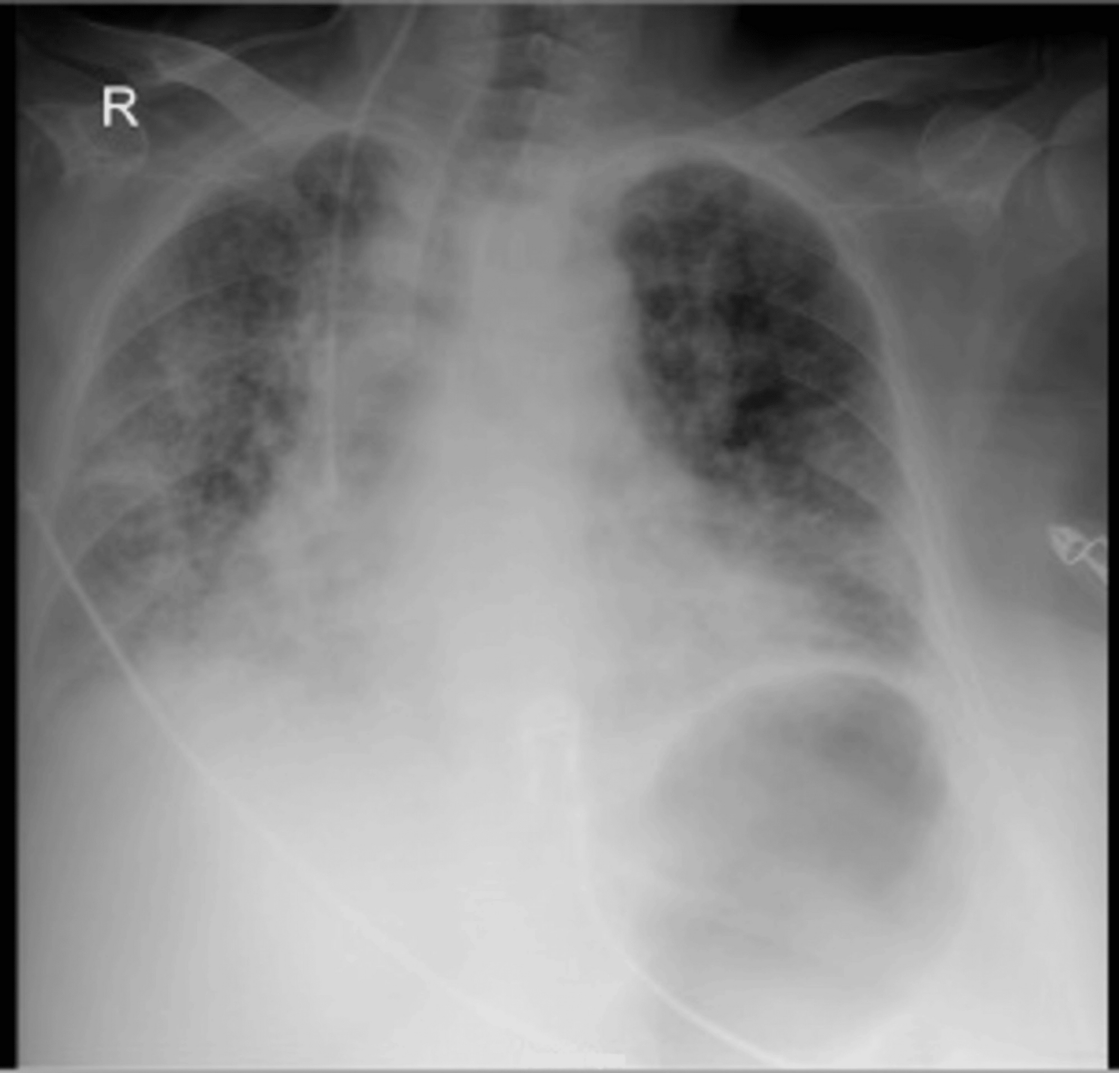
Initial chest X-ray demonstrating bilateral, diffuse micronodular perihilar infiltrates
